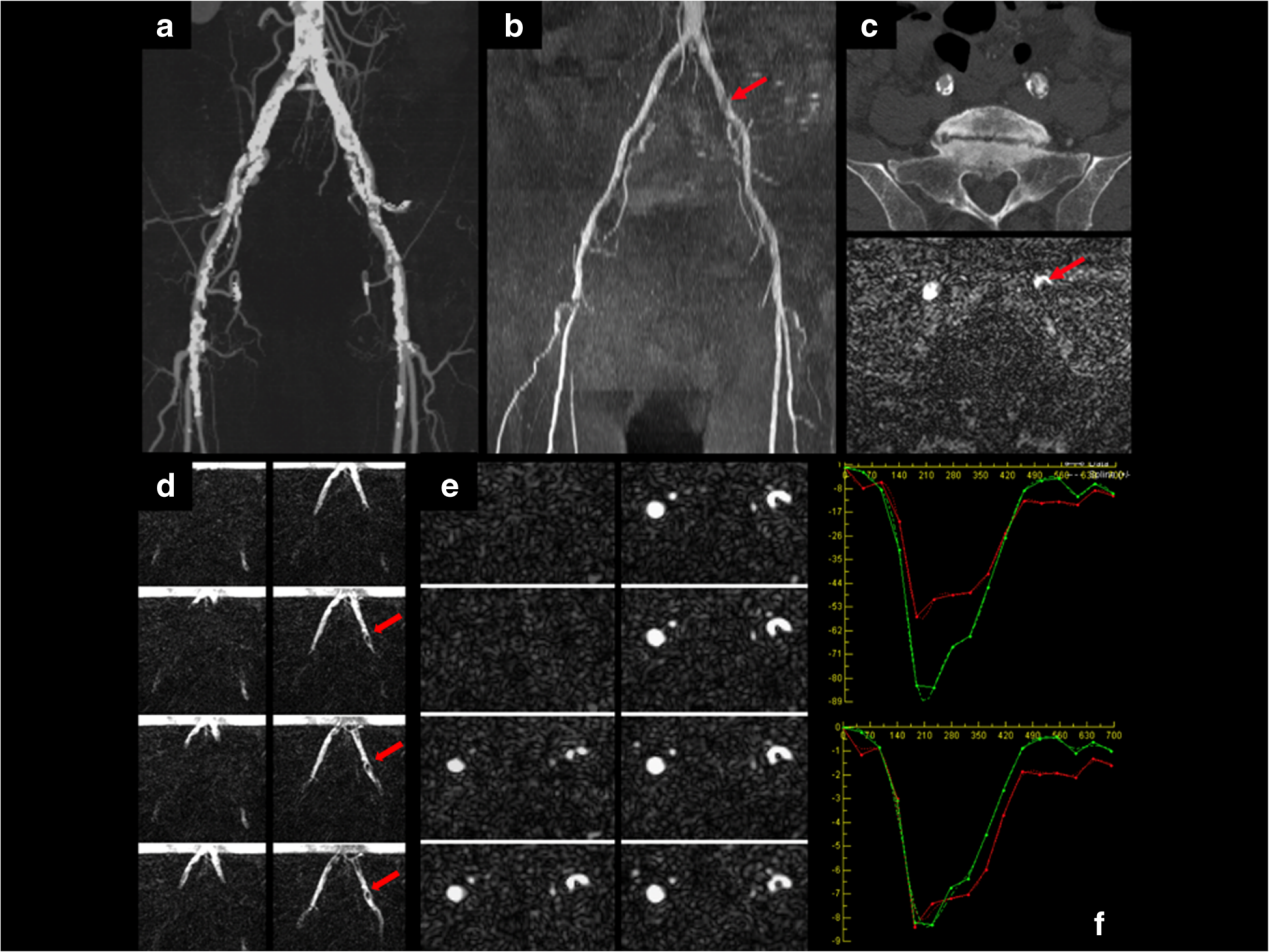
69-year-old male with right leg claudication secondary to occlusion of the proximal right superficial femoral artery. a CTA demonstrates heavily calcified atherosclerotic disease. b QISS MRA. Red arrow = incidental moderate stenosis of the left common iliac artery (CIA). c Axial slice from CTA (top) and QISS MRA (bottom) shows the left CIA stenosis. d Coronal cFASL shows a similar rate of progression of the labeled bolus on the two sides despite the presence of the left-sided stenosis (red arrows). This finding is suggestive that the stenosis is not hemodynamically significant. The left CIA plaque is visible as a partial filling defect in the labeled bolus of arterial blood. e Thin-slice (3-mm) axial cFASL at the level of the stenosis shows the arterial lumen (white) in high contrast to the plaque (dark). f Axial 2DPC at the level of the stenosis shows flow velocity (top) and bulk flow (bottom) for the right (red) and left (green) vessels. 2DPC confirms that the stenosis is not hemodynamically significant since bulk flow is approximately equal between the two sides during the systolic phase of the cardiac cycle, despite the increased peak flow velocity within the stenosis
